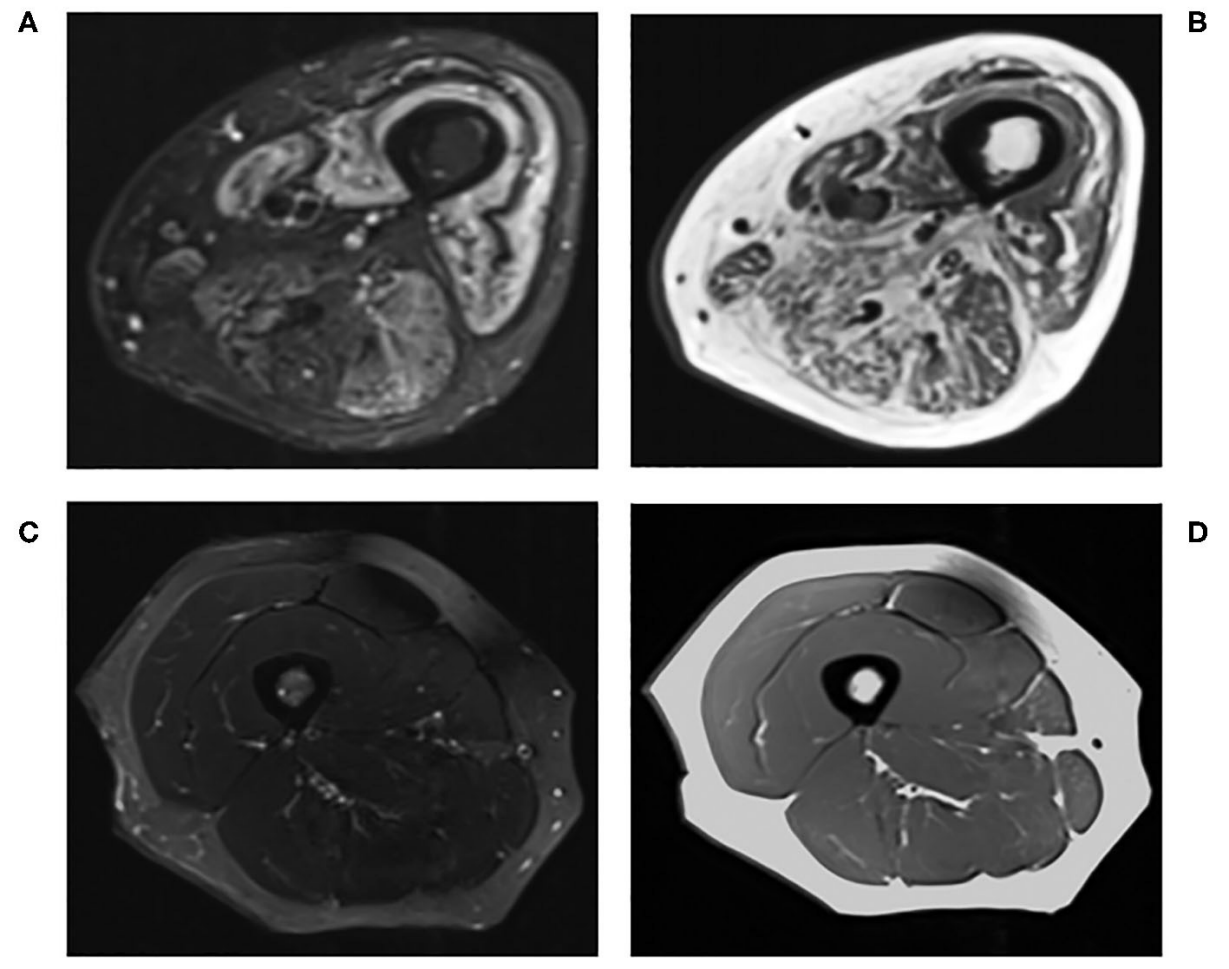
Conventional MRI of the right thigh in (A) T2-STIR and (B) T1-weighted images of a 60-years-old male with active myositis, compared to (C) T2-STIR and (D) T1-weighted images of a 45-years-old healthy female.
